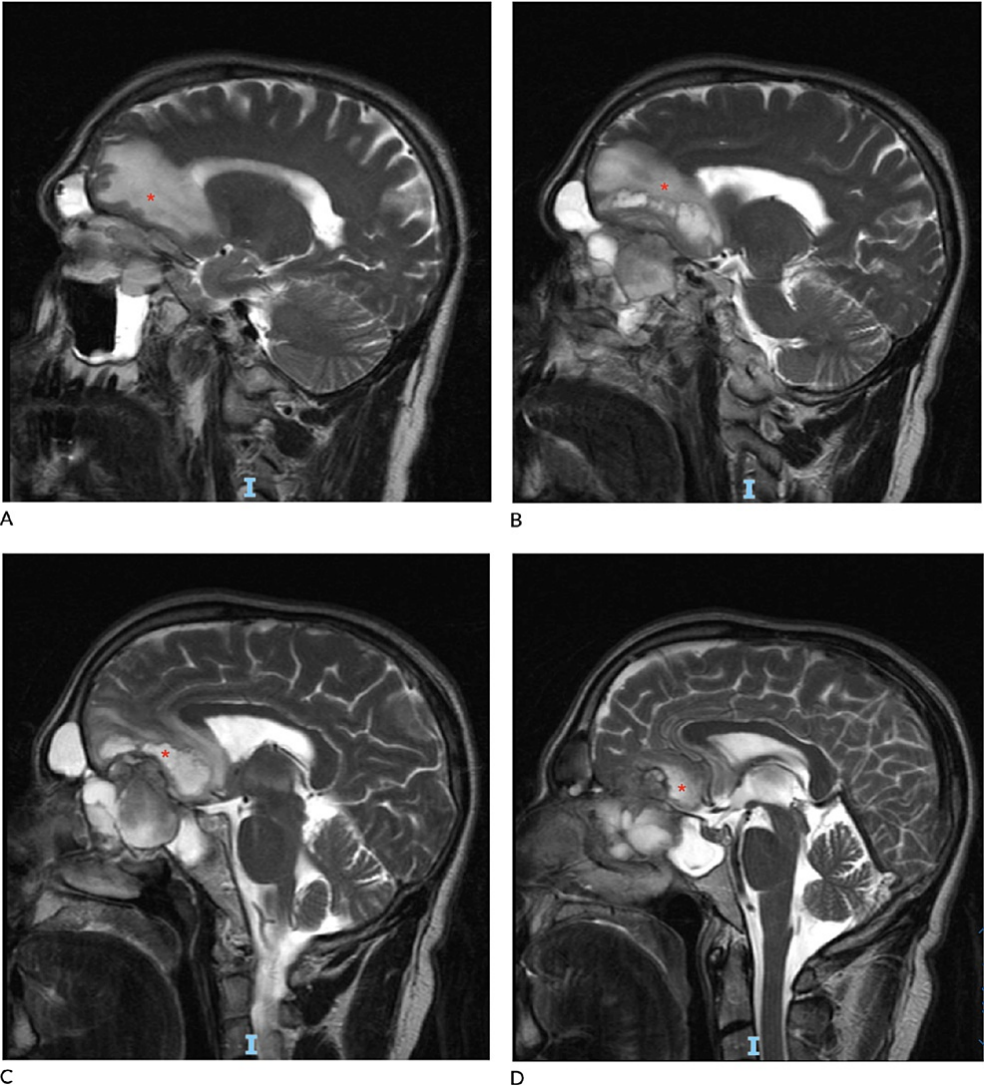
Sagittal MRI of sinonasal mass in ethmoids and extending intracranially, indicated with red asterisk (A-D).
